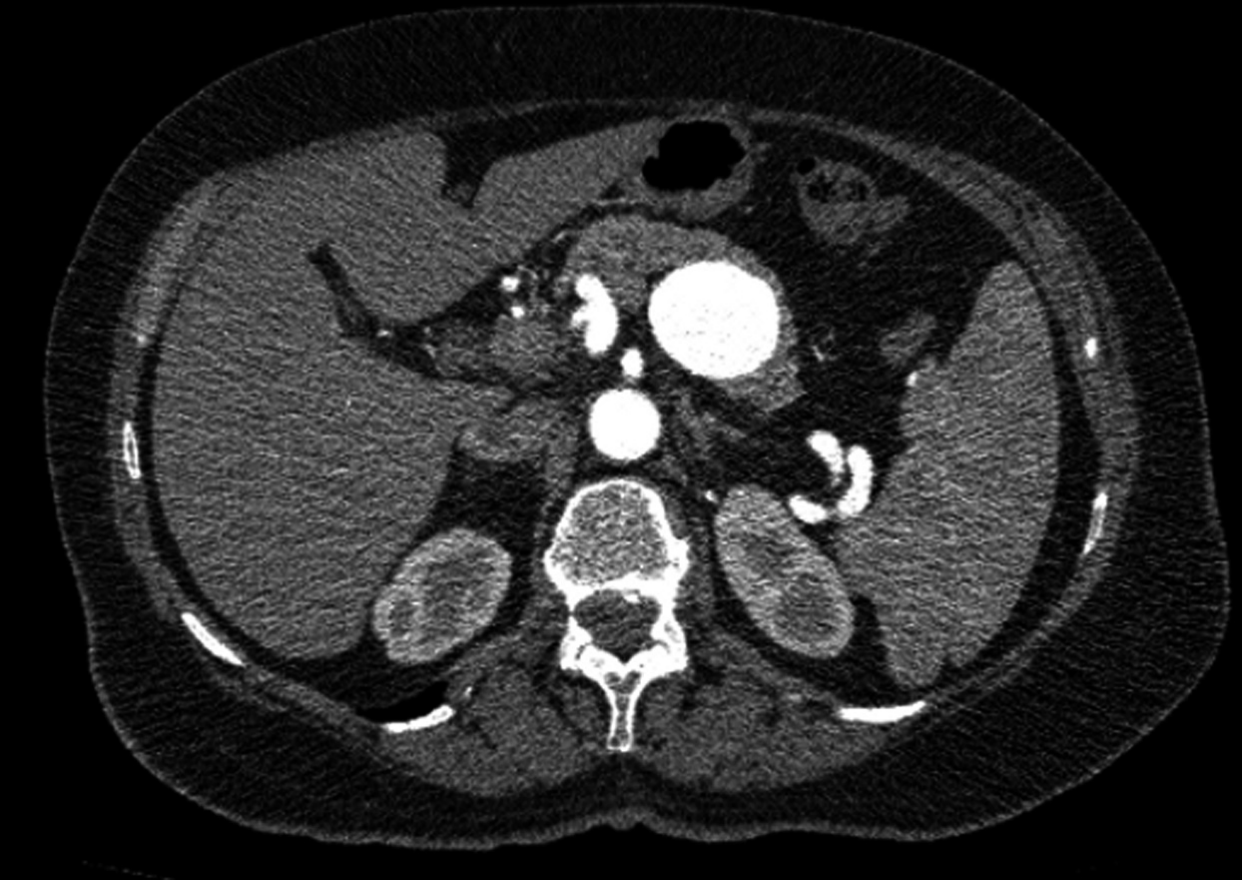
Abdominal CT angiography showing splenic artery aneurysm.
